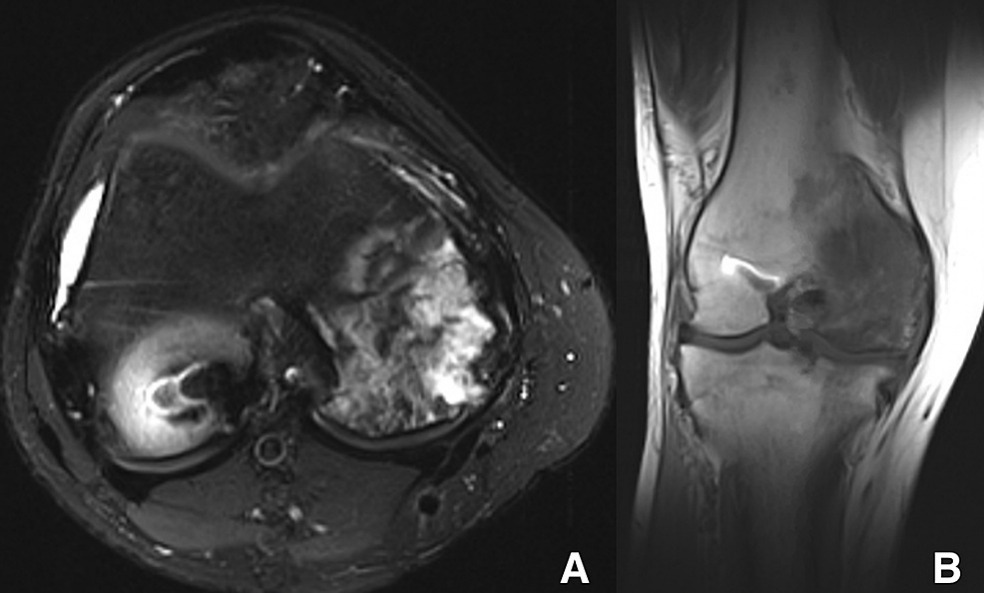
(a) T2-weighted fat-suppressed axial and (b) T1-weighted coronal magnetic resonance images of the right knee of a 29-year-old man (patient three) showing heterogeneity and hyperintensity on T2-weighted signal within the medial femoral condyle.
